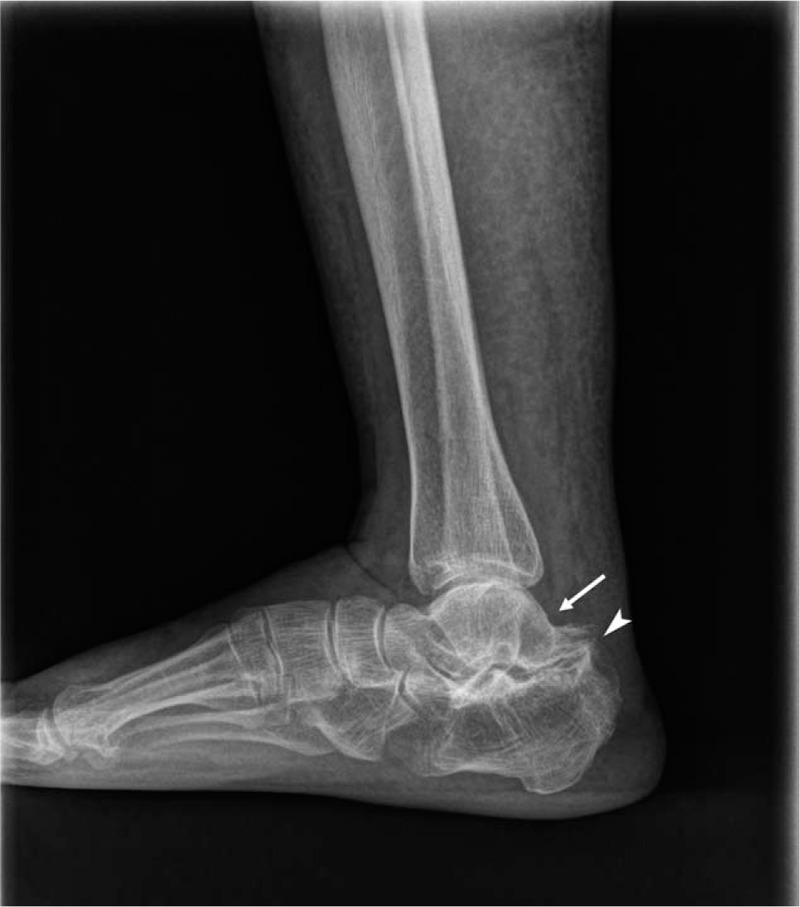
Preoperative plain standing lateral radiography view of the right ankle showing subtalar joint space narrowing and sclerotic bony changes with a deformed C-sign (arrow) and humpback sign (arrowhead).
